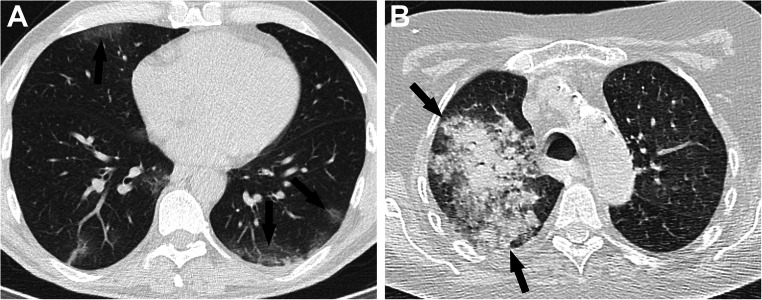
CO-RADS misclassification by the ICOVAI model on the external test dataset. A A 55-year-old patient with small subpleural ground-glass opacities in both lungs (arrows), which consisted of a typical appearance of COVID-19 (CO-RADS 5), later confirmed with RT-PCR. The case was misclassified as negative (CO-RADS 1) by the ICOVAI model. B A 70-year-old patient was admitted to the intensive care unit with lobar pneumonia, atypical appearance for COVID-19 (CO-RADS 2). CT showed infectious consolidation in the right upper lobe (arrows), and increased attenuation due to hypoventilation in the other pulmonary lobes. The case was misclassified as CO-RADS 5 by the ICOVAI model
